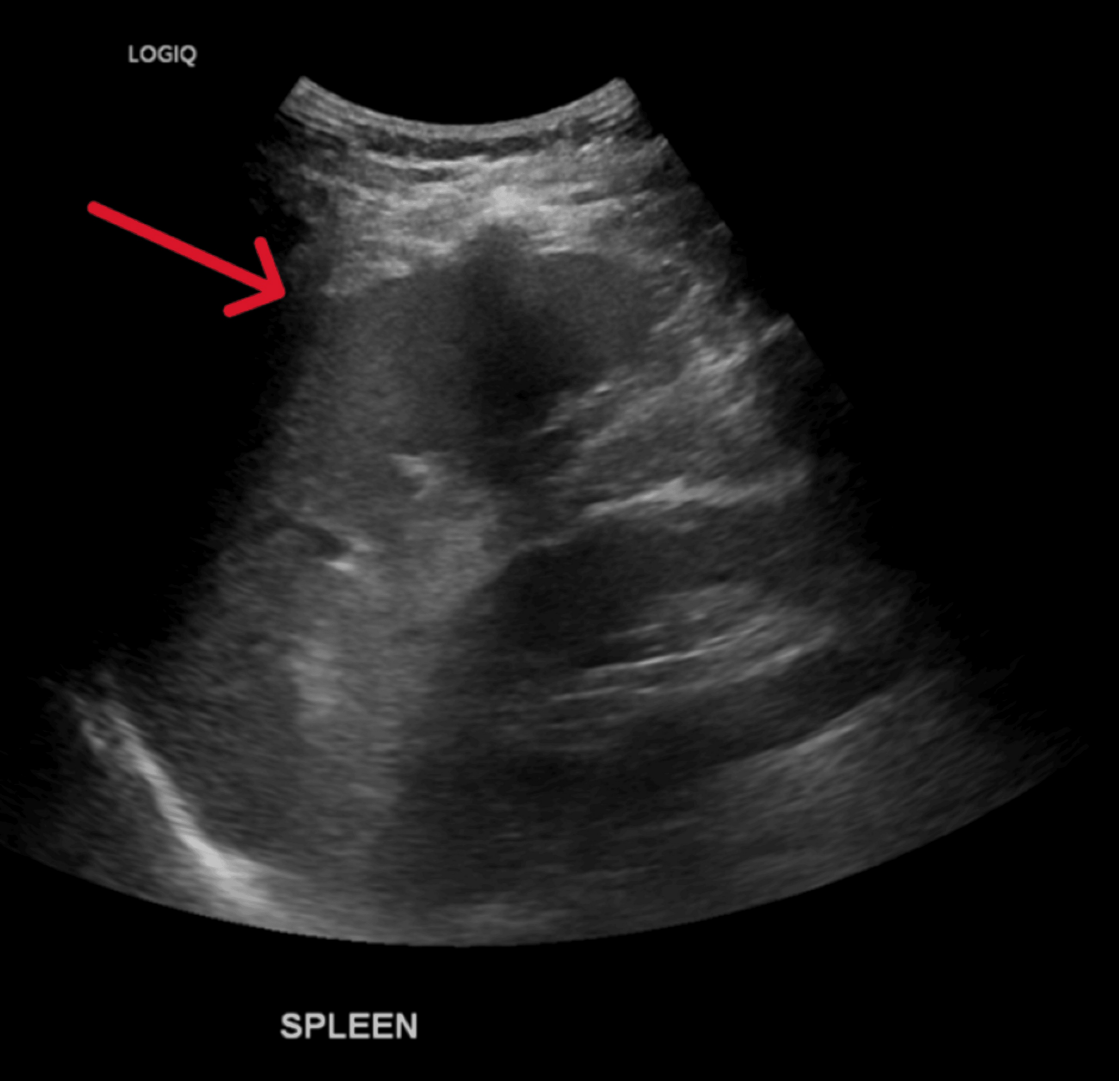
Ultrasound of the abdomen showing mild splenomegaly (red arrow)
